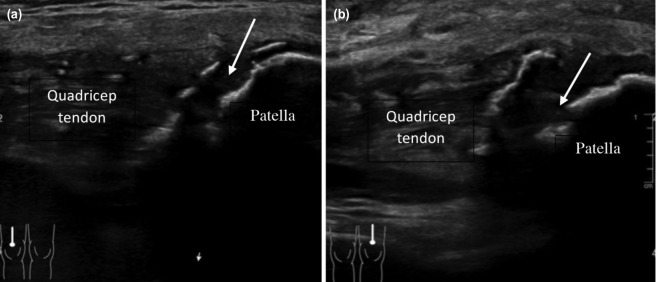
Ultrasound of bilateral knee shows the gap (arrow) in the quadricep tendon which indicates re-rupture, (a) right knee, (b) left knee.
